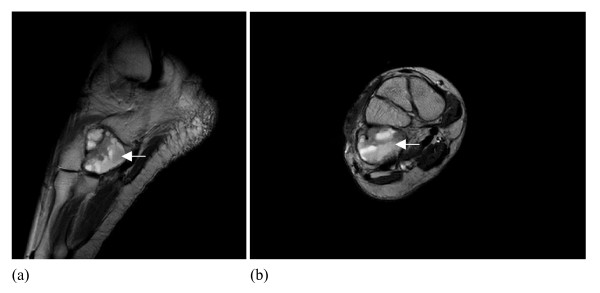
MRI showed cystic areas with fluid filled levels in the right cuboid bone. Mild expansion of the bone was noted. However no cortical break or involvement of other tarsal bones was seen.
